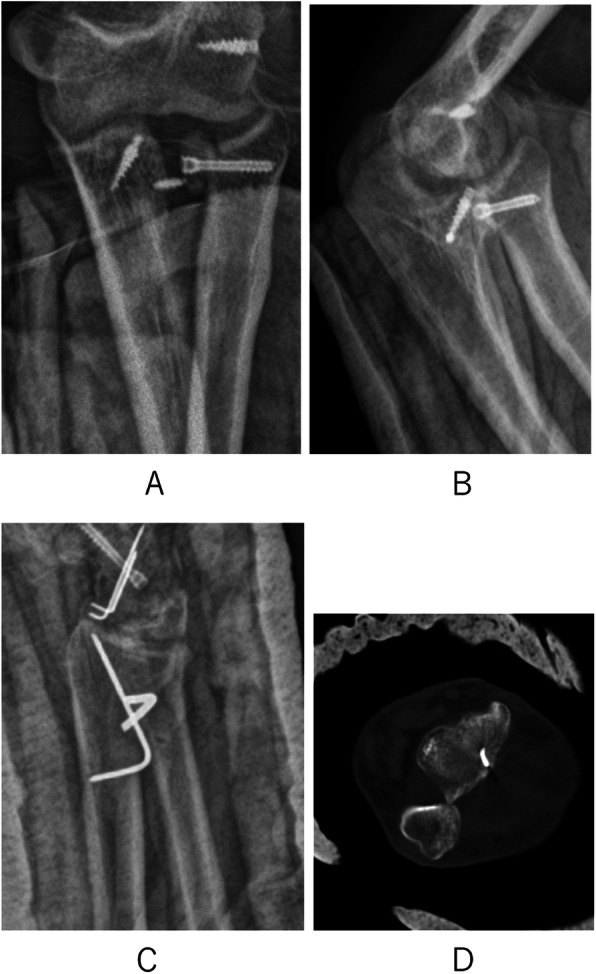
After first surgery radiographs. a-b, elbow joint; c, wrist joint; d, CT: wrist joint
